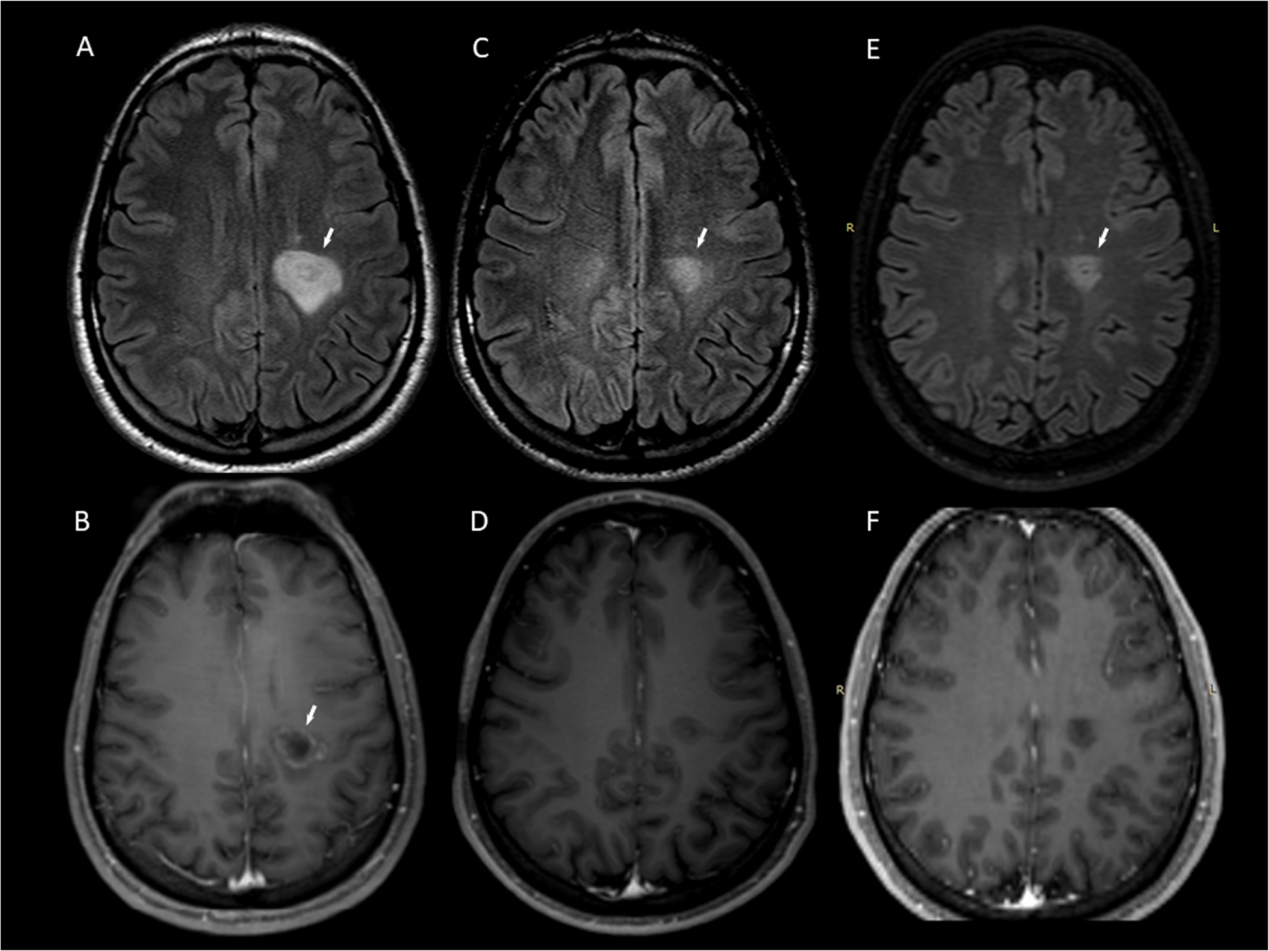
MRI of Case 1 showing BCS-type lesion before and after treatment with cyclophosphamide. Tumefactive BCS-type lesion of the left corona radiata at symptom onset (A) with partial Gd + of the outermost layer (B arrow). A BCS-type lesion with residual ill-defined concentric morphology (C arrow) and no Gd + (D) 15 months from onset, after 6 pulses of cyclophosphamide. BCS-type lesion 5 years from onset (E arrow, F). a, c: FLAIR images. b, d: T1-weighted contrast-enhanced images. e: 3D FLAIR image. f: 3D T1-weighted contrast-enhanced image. BCS: Baló’s concentric sclerosis, Gd+: gadolinium enhancement
